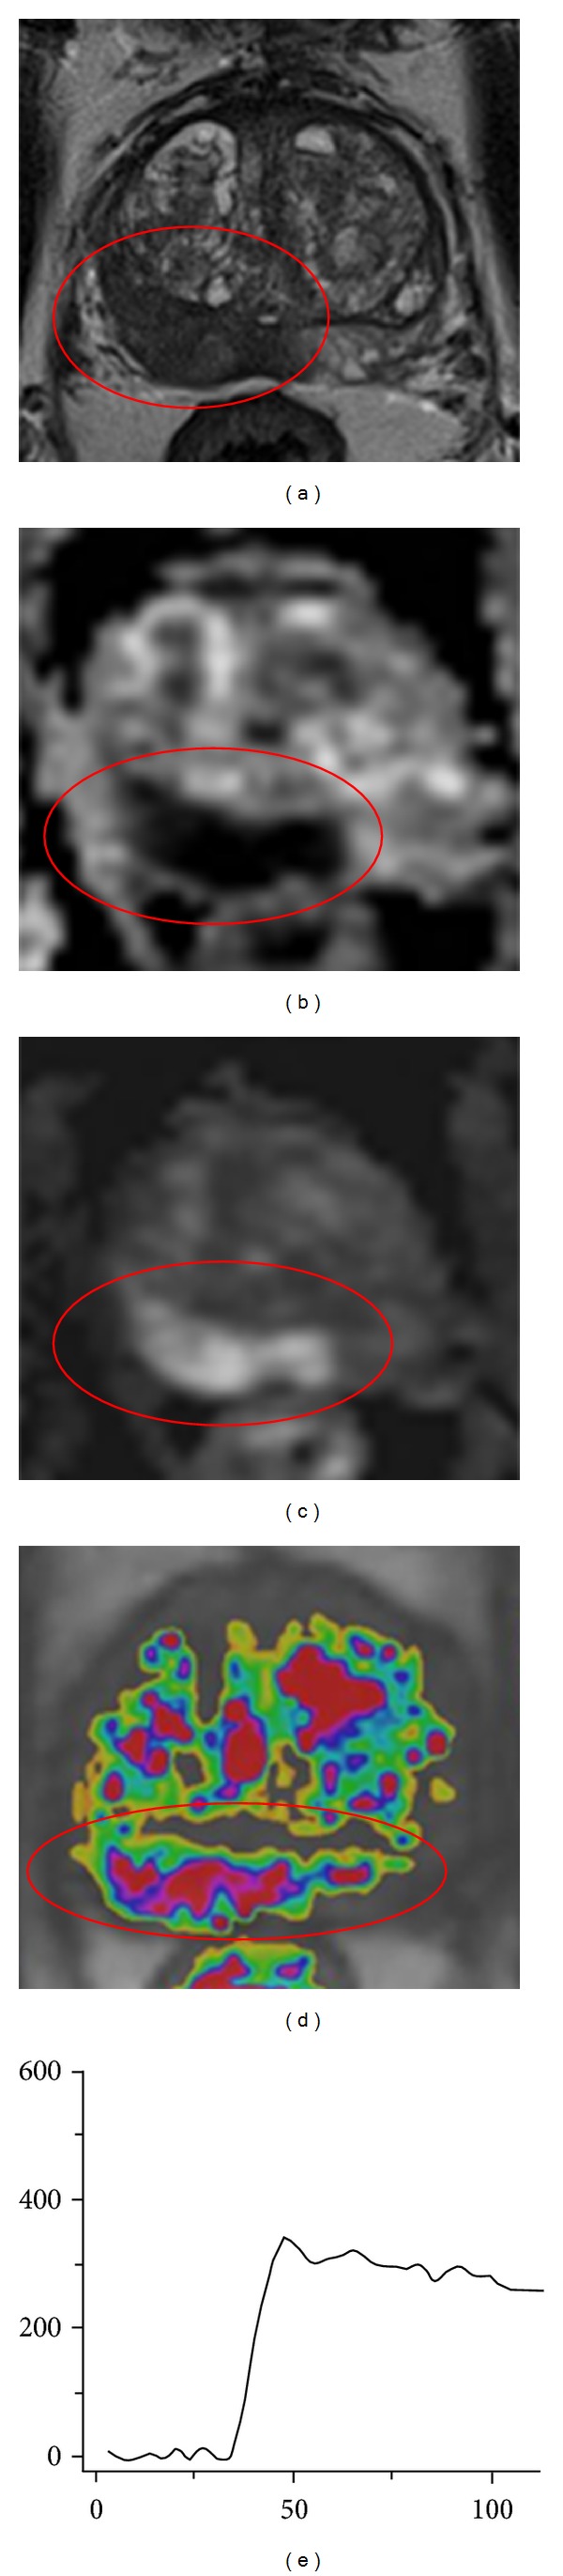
Example of multiparametric MR images of an 83-year-old male, with a PSA level of 10 ng/mL and 0 negative transrectal ultrasound guided biopsy sessions. The encircled lesion scored PI-RADS score 5 on T2W, DWI, and DCE images. Because the lesion is located in the peripheral zone, DWI is the dominant parameter and the final PI-RADS score was PI-RADS 5. (a) Axial T2-weighted image. (b) Axial ADC map. (c) Axial DWI with *b* = 1400. (d) Axial DCE image. (e) Curve of the DCE image.
